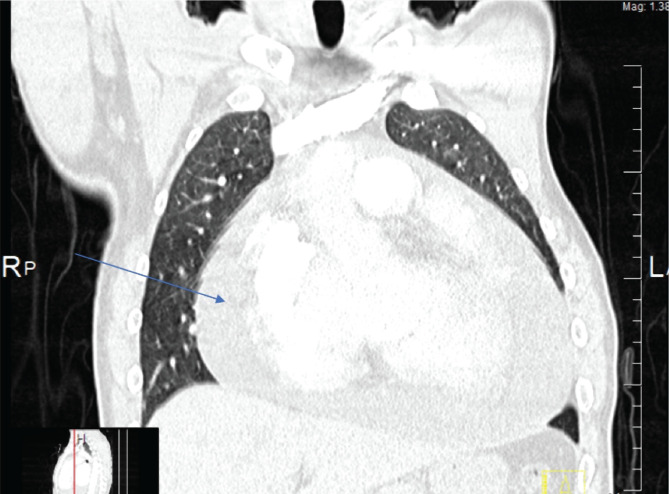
Chest CT coronal view; the arrow indicates the massive pericardial effusion.
